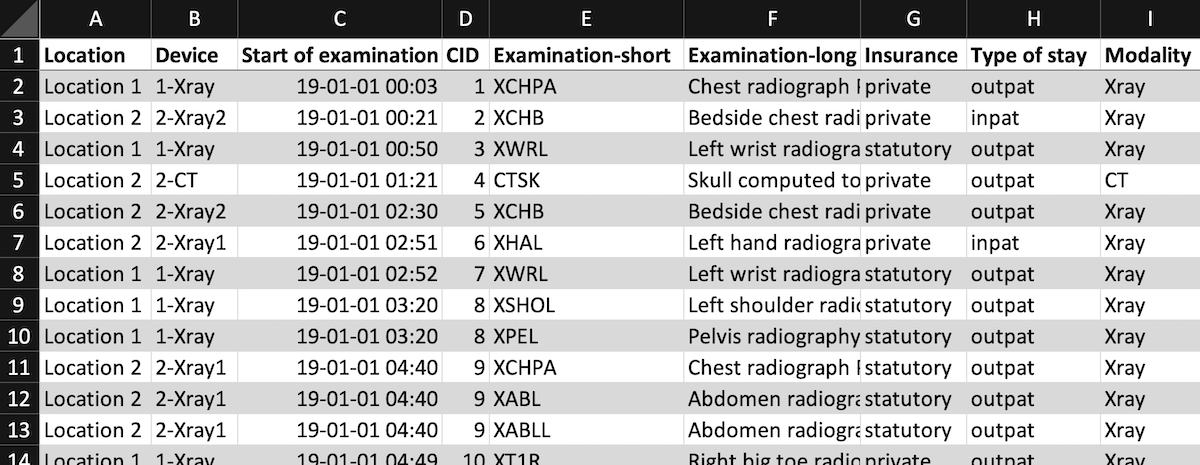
Sample of an RIS Excel export containing information about 1 examination in the radiology department per row and 1 attribute per column. CID: Case identifier; CT: computed tomography; RIS: radiology information system.
